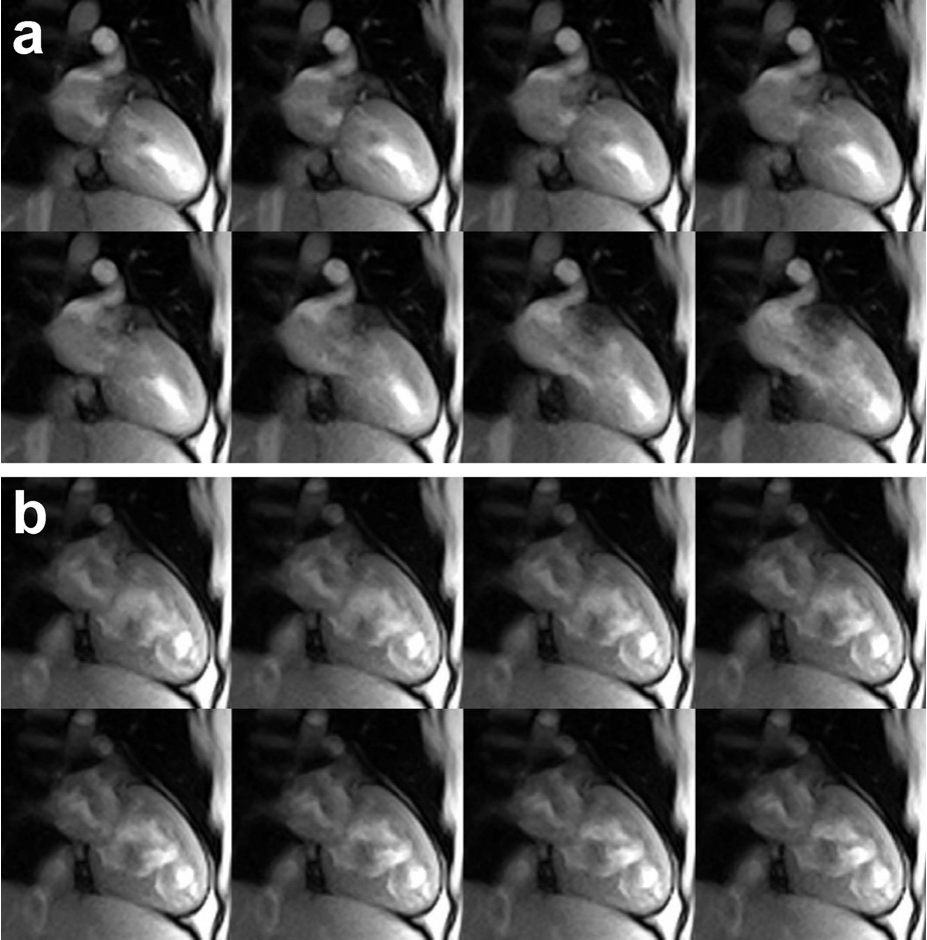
Real-time radial FLASH CMR at 2.0 mm spatial resolution and 22 ms temporal resolution. Consecutive T1-weighted images (spoiled FLASH, TR/TE = 2.0/1.3 ms, flip angle 8°, 11 spokes) of a 2-chamber view at 2.0 mm resolution, 8 mm section thickness, and 22 ms acquisition time. The images depict two 176 ms periods (8 images) of a single cardiac cycle that are 682 ms (31 images) apart: (a) opening of the mitral valve and resulting blood stream into the left ventricle, (b) turbulent flow leading to a vortex at the apex of the left ventricle. They were selected from a respective real-time CMR movie reconstructed by nonlinear inversion (see Additional File 2) and zoomed by a factor of 1.5.
